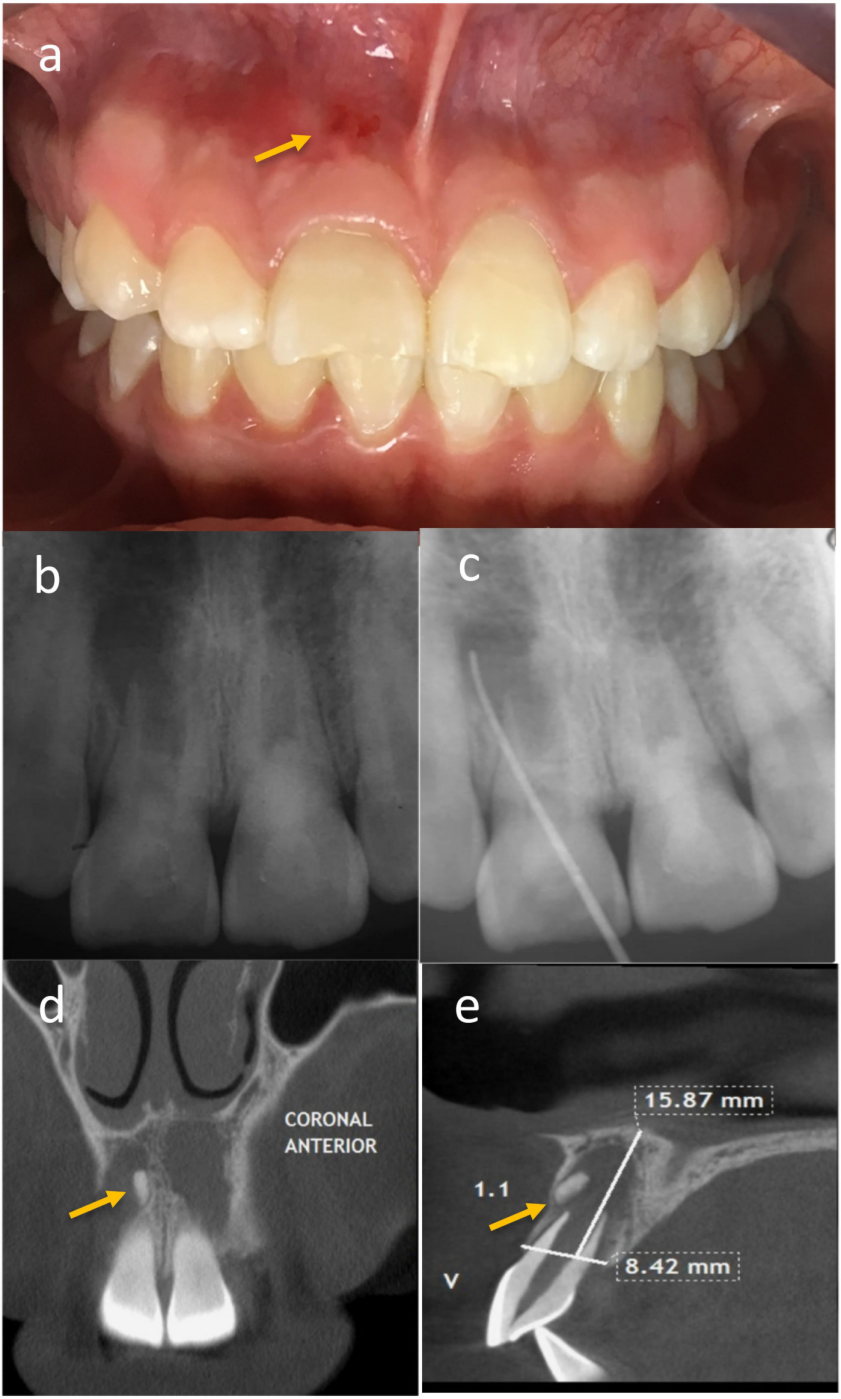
(a) Patient reported severe pain in tooth 1.1, and clinically, an erythematous area in the mucosa with pain on percussion and palpation of the vestibular fold was found. (b) A control radiograph was taken and the patient was maintained under observation. (c) An active sinus tract related to tooth 1.1 was observed, and fistula catheterization was performed. (d,e) A possible dental formation associated with tooth 1.1.
